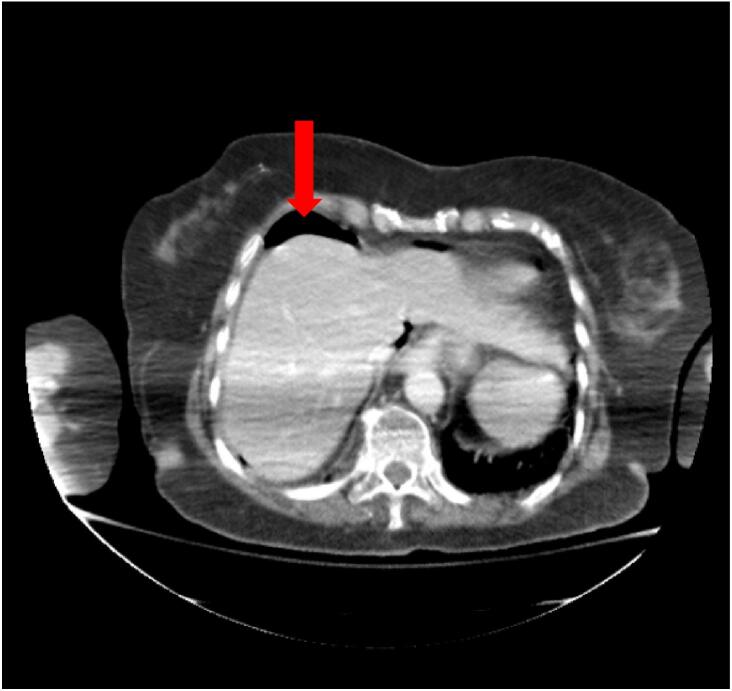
Computed tomography of the abdomen and pelvis revealing pneumoperitoneum (red arrow). (For interpretation of the references to colour in this figure legend, the reader is referred to the web version of this article.)
